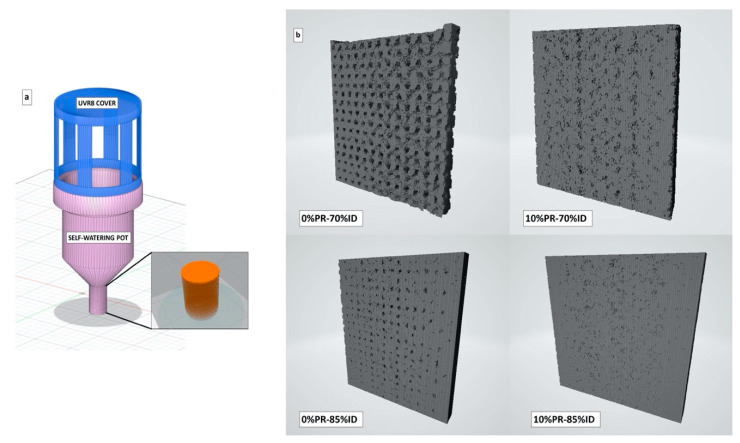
3D model of smart irrigation prototype (a) and a 3D model reconstruction obtained from X-ray tomography analysis of the permeable part 3D printed at different ID percentage (b).
